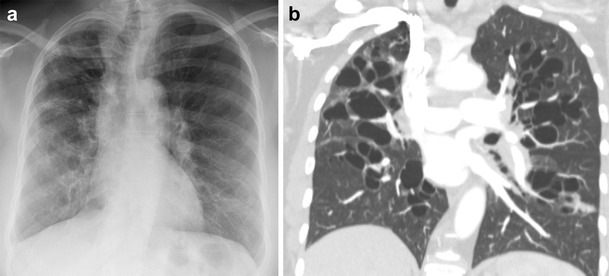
Williams-Campbell syndrome. a Frontal chest x-ray demonstrating bilateral architectural distortion and coarse interstitial markings with cystic appearing regions in the lower lobe consistent with the scarring and diffuse saccular bronchiectasis seen in Williams-Campbell syndrome. b Coronal CT imaging demonstrating the severe bilateral cystic bronchiectasis of the subsegmental (4th-6th generation) bronchi commonly seen in Williams-Campbell syndrome
